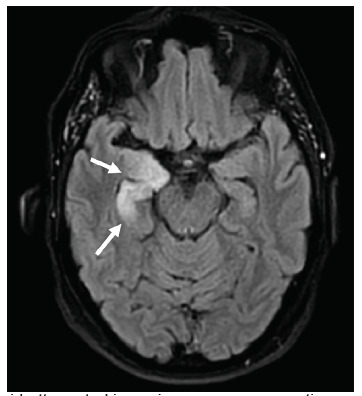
Fluid-attenuated inversion recovery magnetic resonance imaging demonstrating asymmetric swelling and edema within the right hippocampus (white arrows) suggestive of limbic encephalitis.
